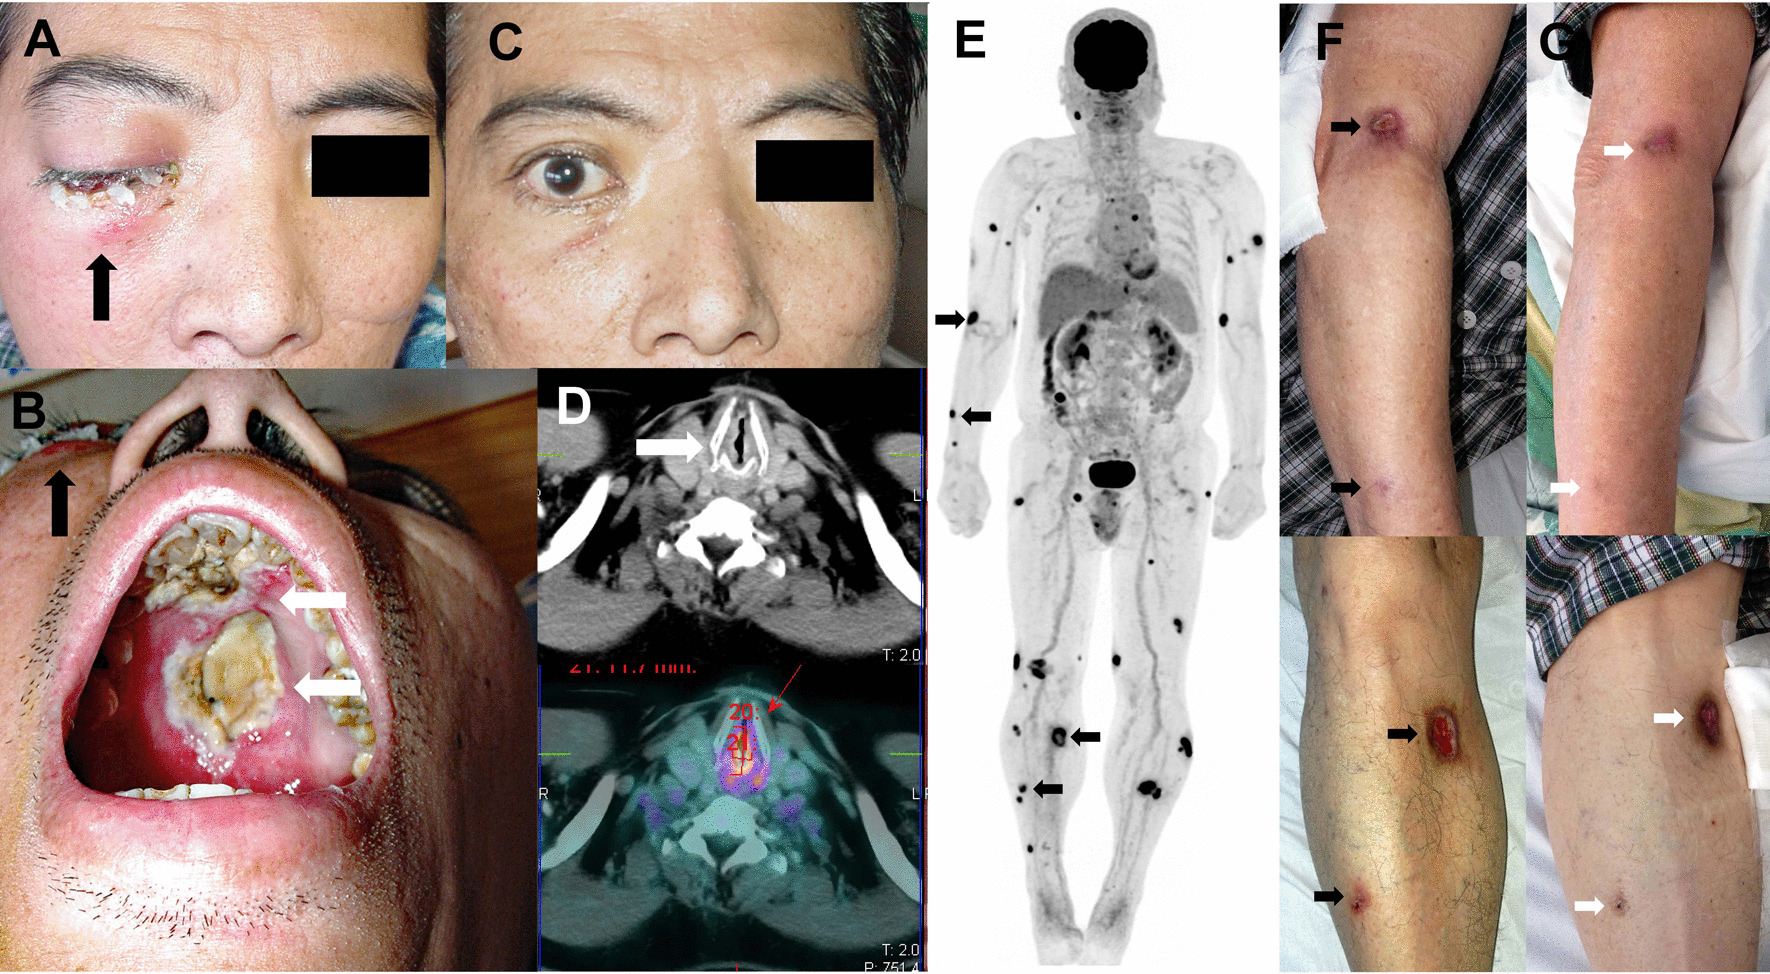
Clinical subtypes of NK/T-cell lymphomas. a Nasal NK/T-cell lymphoma with superior invasion into the right orbit, leading to extensive swelling and scabbing (arrow). b Same case with right orbital invasion (black arrow). Inferior invasion resulted in extensive necrosis and almost complete destruction of the hard palate (white arrows). The ensuing perforation of the hard palate would lead to a communication between the nasal and oral cavities, giving rise to the classical “lethal midline granuloma”. c Same patient about two weeks after commencement of the first cycle of the SMILE regimen. There was rapid and complete resolution of the right orbital swelling and scabbing. d Another case of upper aerodigestive tract NK/T-cell lymphoma. There was extensive involvement of the subglottis (arrow), which was markedly hypermetabolic on positron emission tomography computed tomography (PET/CT). Note that the larynx was reduced to a mere slit, causing nearly fatal airway obstruction that necessitated emergency tracheostomy. e A case of apparent non-nasal NK/T-cell lymphoma with extensive skin involvement, which on PET/CT scan was shown as numerous hypermetabolic cutaneous deposits (arrows). Examination of the nasopharynx did not show any obvious lesion. However, blind biopsies showed nasopharyngeal involvement, rendering this case indistinguishable from nasal NK/T-cell lymphoma with extensive cutaneous metastases. f Cutaneous lesions of the same case, with arrows indicating deposits corresponding to those shown by arrows on the PET/CT (e). g After the first cycle of an asparaginase-containing regimen, showing complete healing of the skin lesions (arrows)
